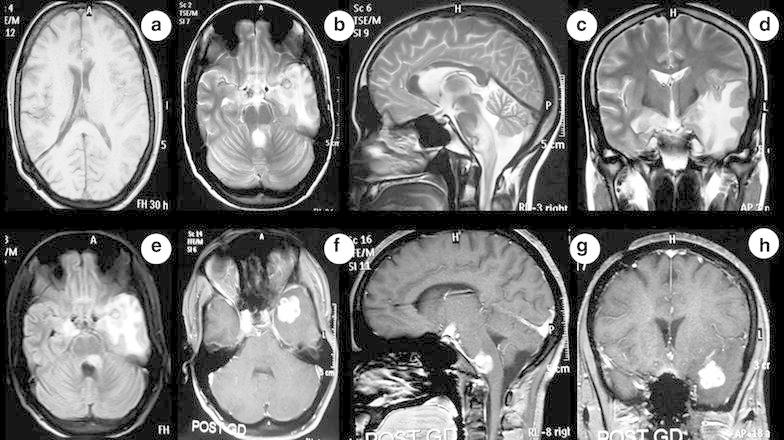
MRI of brain shows multiple intraparenchymal lesions involving brainstem and anterior temporal lobe. These lesions are isointense to grey matter on T1-weighted (a), hyperintense on T2-weighted (b, c, d) and fluid attenuated inversion recovery (FLAIR) (e) images. There is no central hyperintensity on T2-weighted image. Surrounding perilesional vasogenic edema is present. Gadolinium-enhanced T1-weighted images (f, g, h) shows multiple conglomerated ring enhancement. Ventriculoperitoneal shunt in situ is seen in right lateral ventricle
